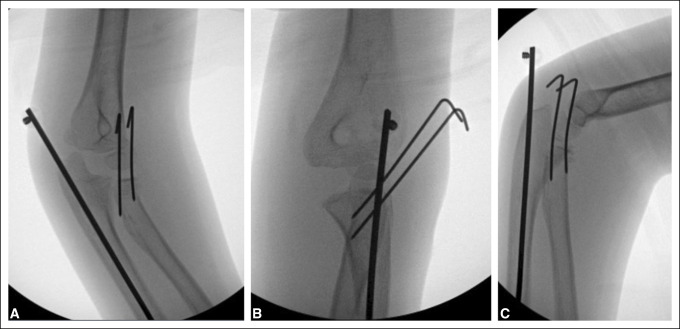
Radiographs of intraoperative fluoroscopy showing reduction and fixation of fractures.
